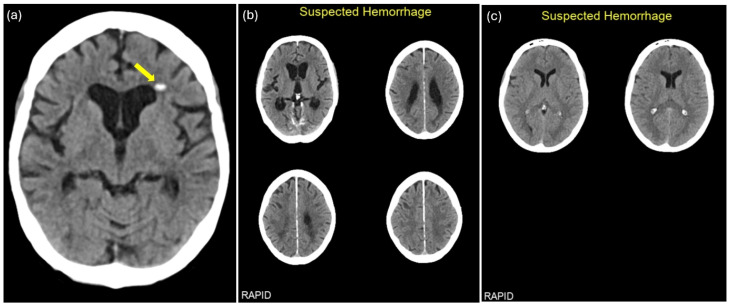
Examples of discrepancies between RAPID ICH identifications and ground-truth labeling presented on 5 mm reconstructed axial non-contrast head computed tomography (NCCT) images. (a) Example of a false-negative RAPID ICH identification where a small intraparenchymal hemorrhage (yellow arrow) in the left frontal lobe was not recognized; (b) example of a false-positive RAPID ICH identification probably related to dural thickening; (c) example of a false-positive RAPID ICH identification probably related to choroid plexus calcifications.
